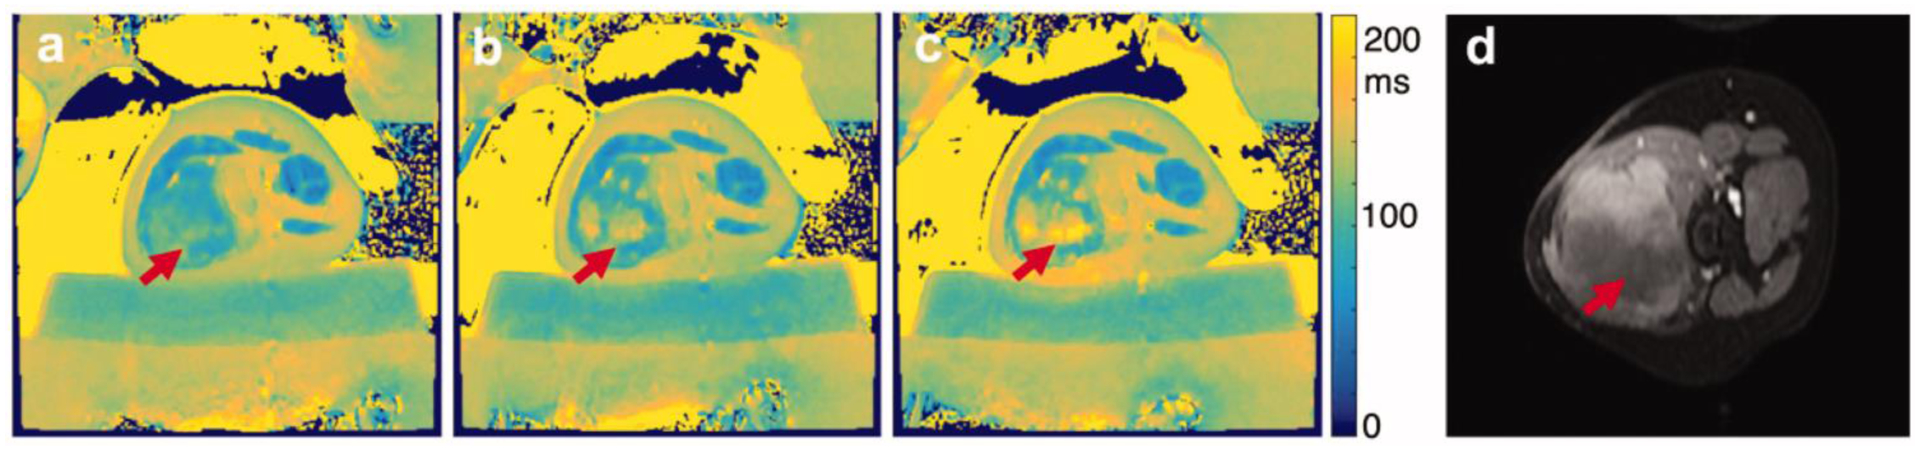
MRI T2 mapping pre and postthermal HIFU ablation desmoid tumors. Pre and postaxial T2 maps (a–c) of one slice for a thermal HIFU treatment. (a) demonstrates the tissue before sonication treatment. (b and c) demonstrate increased T2 value over the course of the treatment duration. (d) postcontrast image of same location. Patient was repositioned, but the arrows indicate the ROI. T2 maps were generated in MATLAB. Figure adapted from [11].
